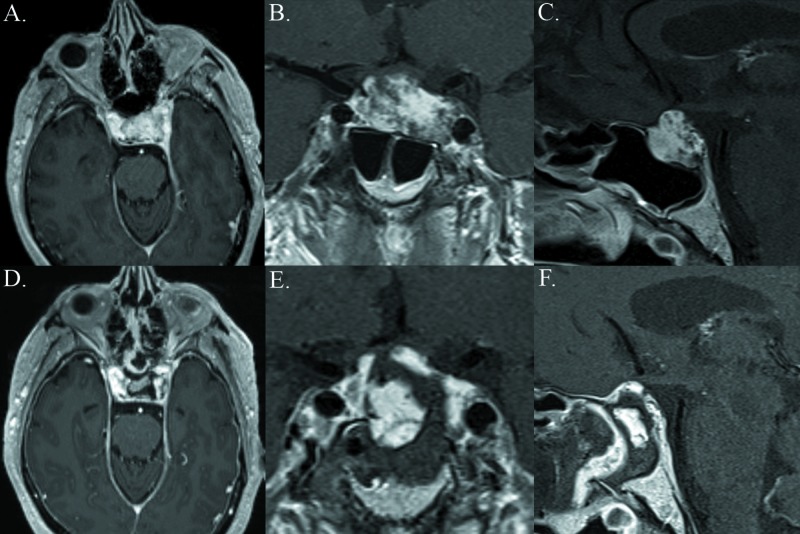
Gross total resection of the sellar cavernous hemangioma Upper: Preoperative axial, coronal, and sagittal T1-weighted MRI studies obtained after the administration of Gd, demonstrating a sellar contrast-enhancing recurrent mass measuring 2.7 cm x 1.9 cm x 1.6 cm extending into the left cavernous sinus Lower: Postoperative axial, coronal, and sagittal T1-weighted MRI studies obtained after gross total resection with EES. Fat graft was used under the vascularized nasoseptal flap. MRI: magnetic resonance imaging; EES: endoscopic endonasal surgery
